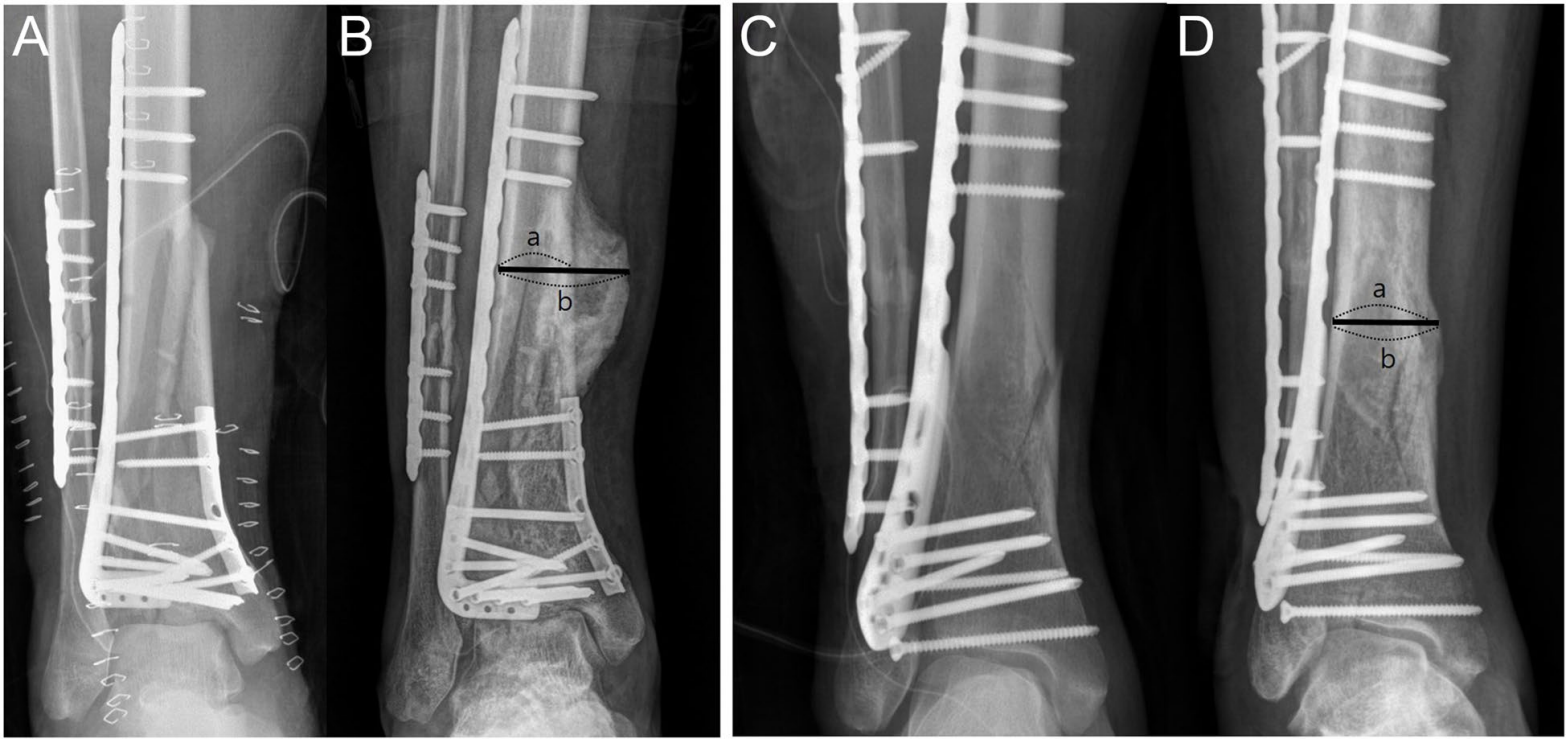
A, B AP radiographs of a patient with traumatic brain injury at immediate post-operative and at 6 months follow-up. C, D AP radiographs of a patient without traumatic brain injury at immediate post-operative and at 3 months follow up. Callus ratio measuring method by Spencer = b/a
